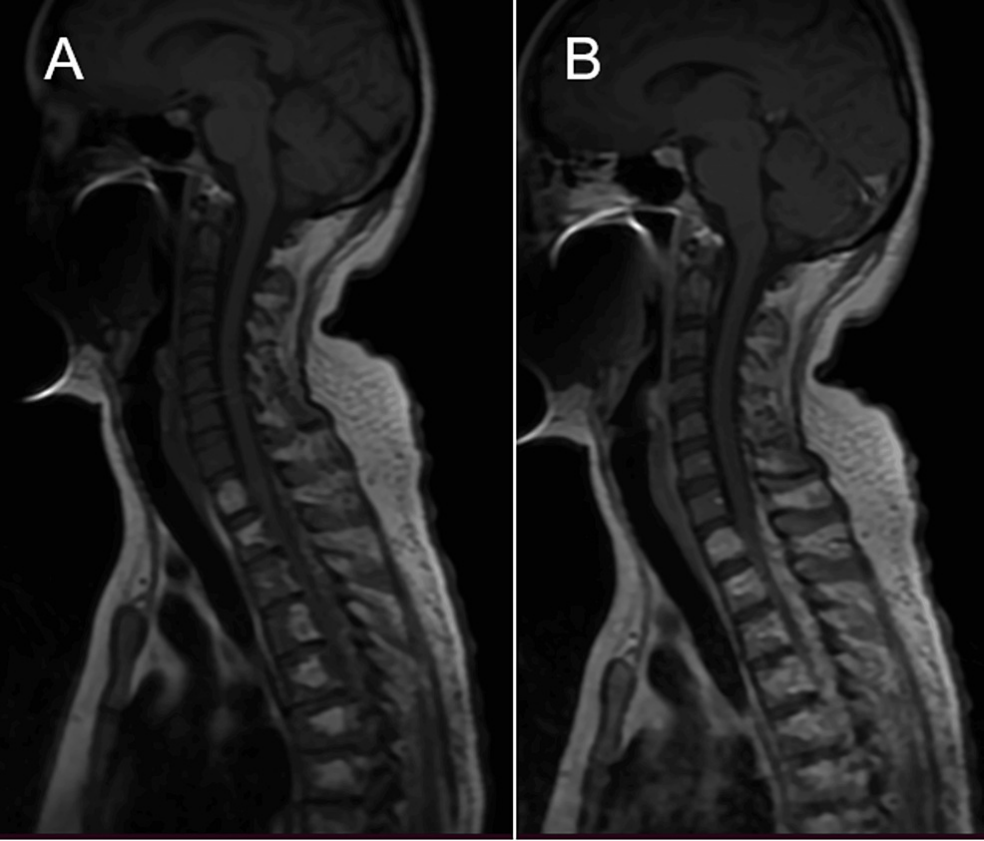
Sagittal magnetic resonance imaging of the cervical and upper thoracic spinal cord (A) T1-weighted image showing predominantly hyperintense extradural mass at the posterior margin of the spinal cord, caudally from C7. (B) T1 using a gadolinium-based contrast agent showing the same lesion with homogeneous contrast enhancement and the involvement of vertebral bodies from C8
